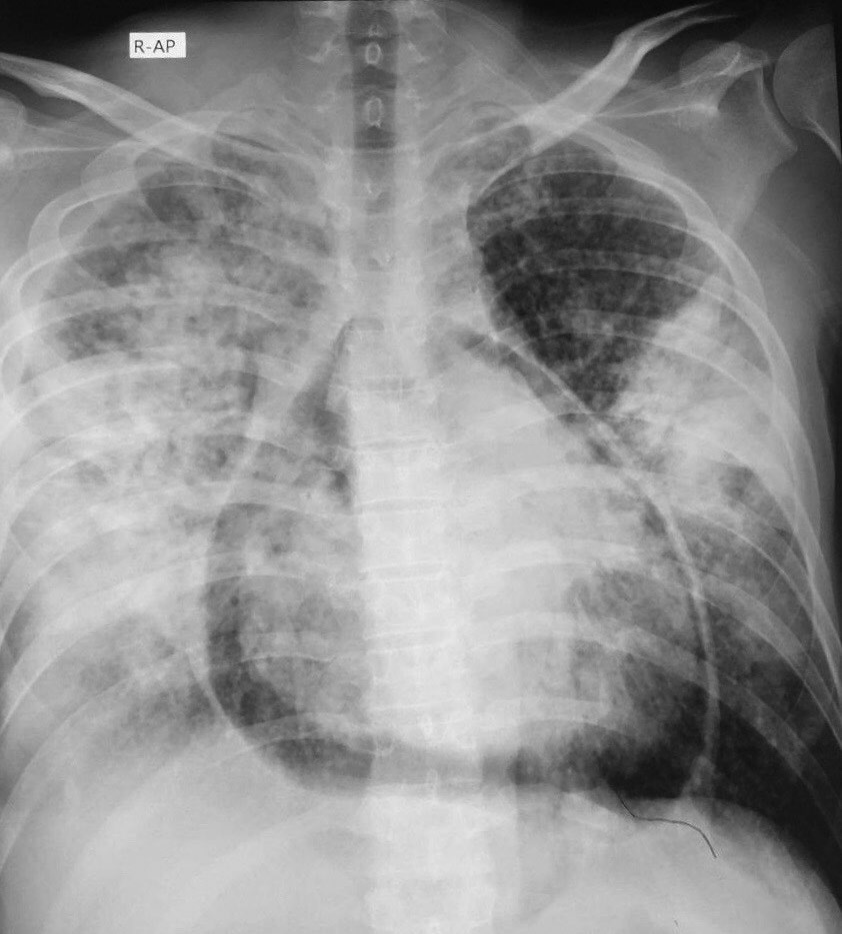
Antero‐posterior chest radiograph shows an air space surrounding the heart consistent with pneumopericardium. In addition, there are bilateral infiltrates in both lung fields
